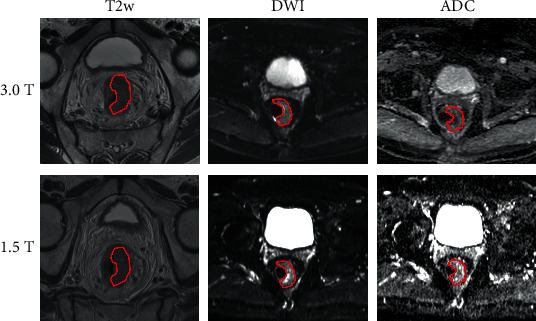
The picture shows manual segmentation of tumor region of interest in three different sequences (T2w, DWI, and ADC maps) on both field strengths, at the same slice.
